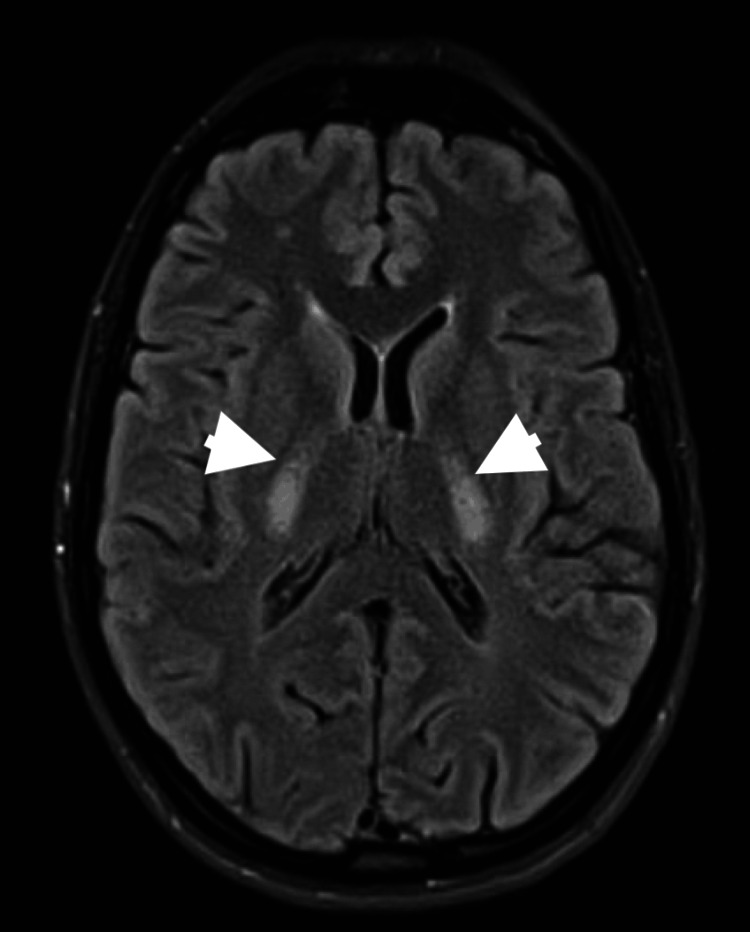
Axial MRI fluid-attenuated inversion recovery (FLAIR) sequence demonstrating symmetric hyperintensities in the bilateral internal capsules (arrowheads).
